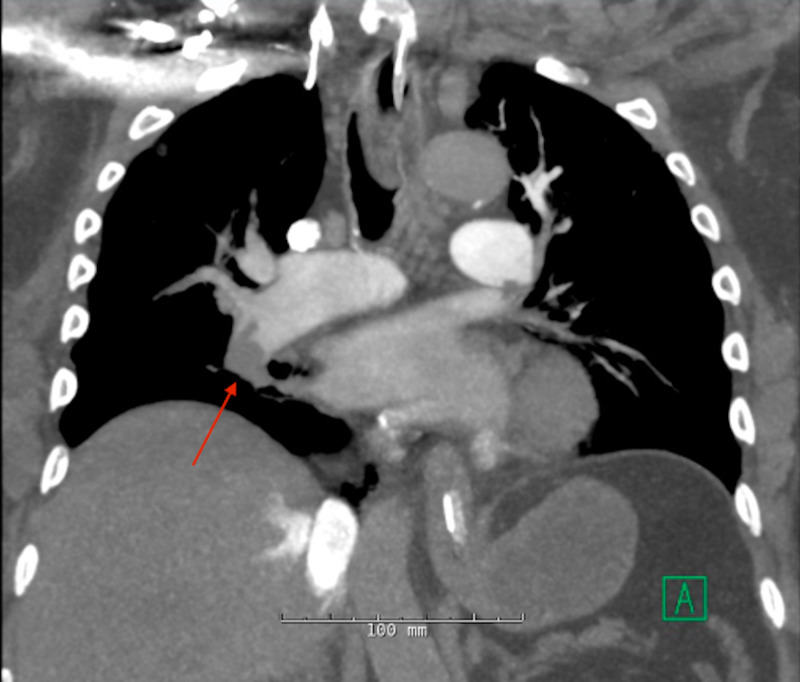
Initial CT angiography of the lungs in the setting of hypoxia shows a large clot burden in the right pulmonary artery (red arrow).
